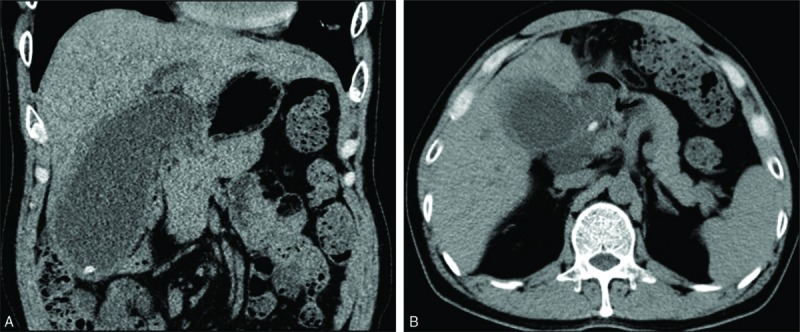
Computed tomography (CT) image showing (A) the gallbladder stone and (B) the combine bile duct stone. CT = computed tomography.
